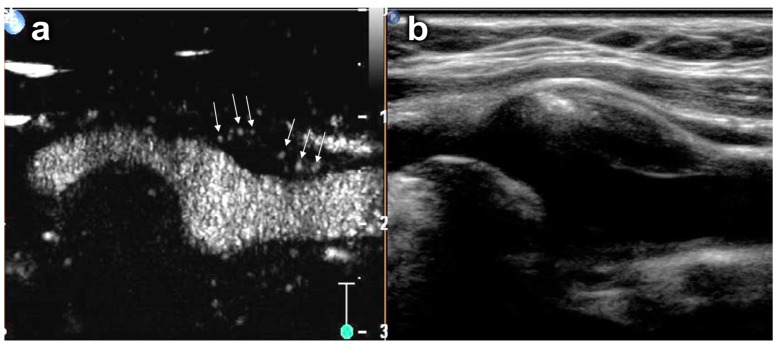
Contrast-enhanced ultrasound (CEUS) imaging of plaque neovascularization in a carotid plaque. (a) Carotid artery with intraplaque neovascularization on CEUS. The arrows denote microbubbles within neovessels in a plaque at the origin of the internal carotid artery; (b) Corresponding B-mode ultrasound image. Reproduced from [71] with permission.
